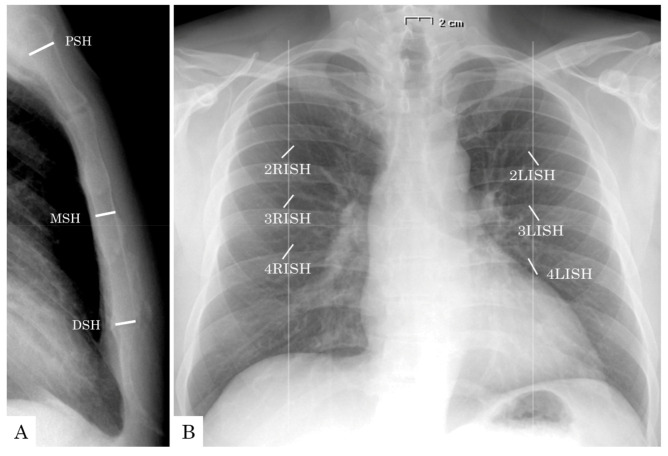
Perioperative measurements at chest X-ray. (A) PSH: proximal sternal height; DSH: distal sternal height; MSH: midsternal height. (B) 2LISH: 2nd left intercostal space height; 3LISH: 3rd left intercostal space height; 4LISH: 4th left intercostal space height; 2RISH: 2nd right intercostal space height; 3RISH: 3rd right intercostal space height; 4RISH: 4th right intercostal space height.
